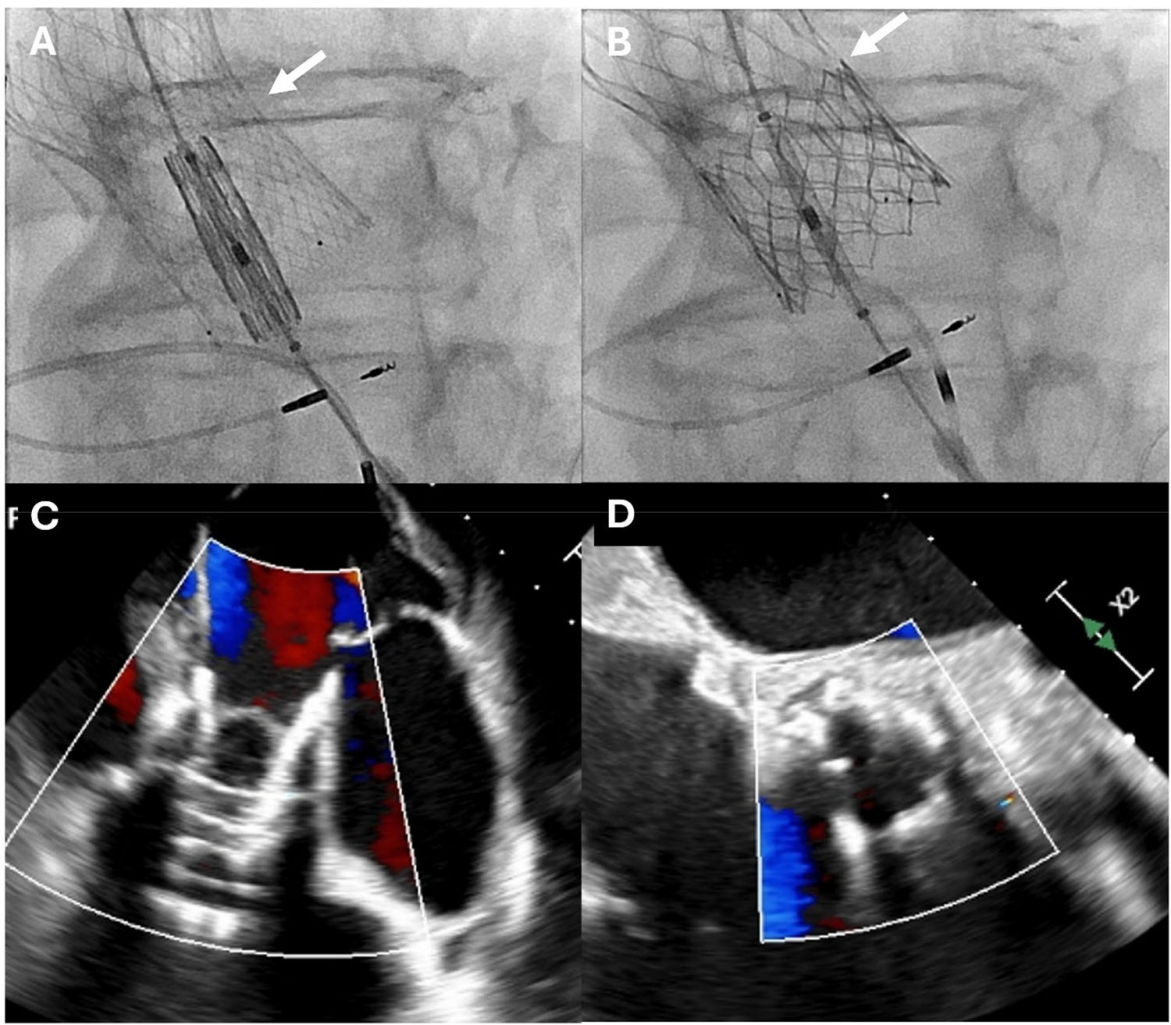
No paravalvular leak after deployment of 26 mm Sapien 3 Ultra Valve in the 26 mm Evolut FX+ valve. The 26 mm Sapien 3 Ultra valve was positioned with the outflow at node 6 of the Evolut (A) and was slowly deployed at nominal inflation volume to full expansion at node 6 (B) (white arrows). TEE color Doppler of the aortic valve in deep transgastric (C) and mid‐esophageal aortic valve short axis views (D) showed no PVL. [Color figure can be viewed at wileyonlinelibrary.com]
